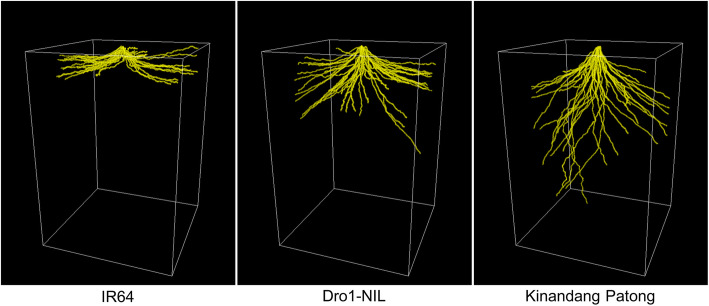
Three-dimensional rendering of IR64, Dro1-NIL, and KP roots. The CT images were processed and vectorized by applying RSAvis3D and RSAtrace3D, respectively. The cube indicates (width) 180 mm × (depth) 180 mm × (height) 258 mm
